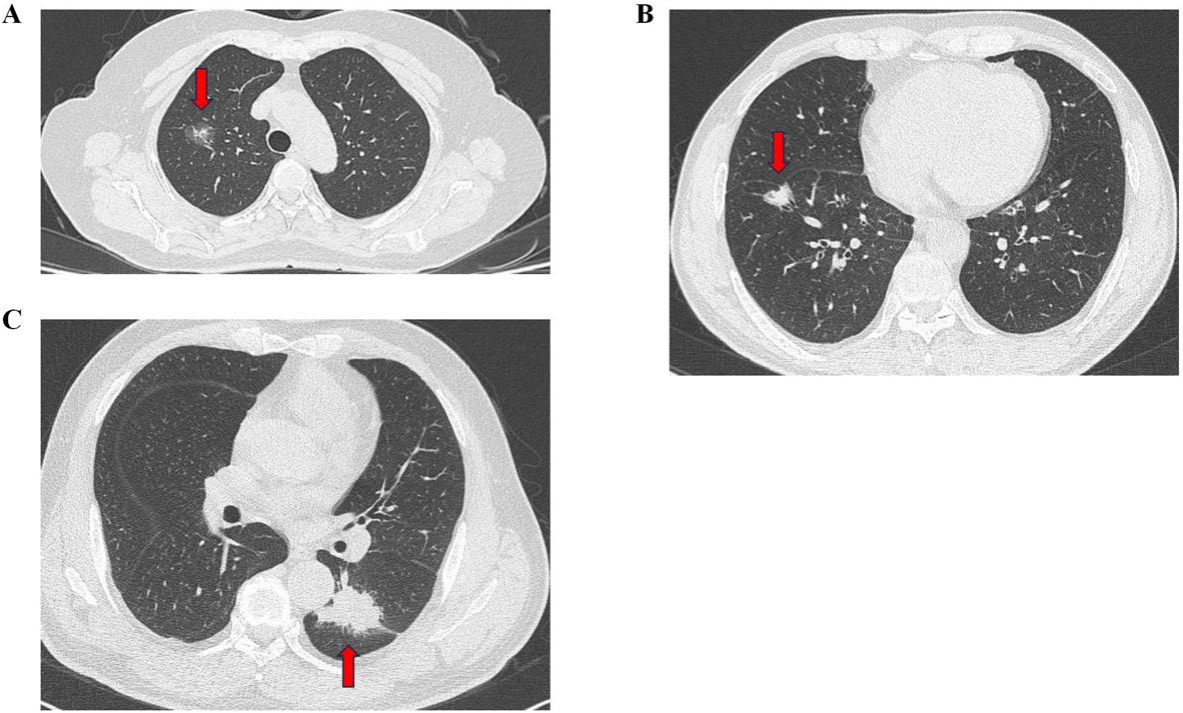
Representative CT images of PD-L1 expression in NSCLC. (A) Negative, (B) TPS 1–49%, and (C) TPS ≥ 50%.
